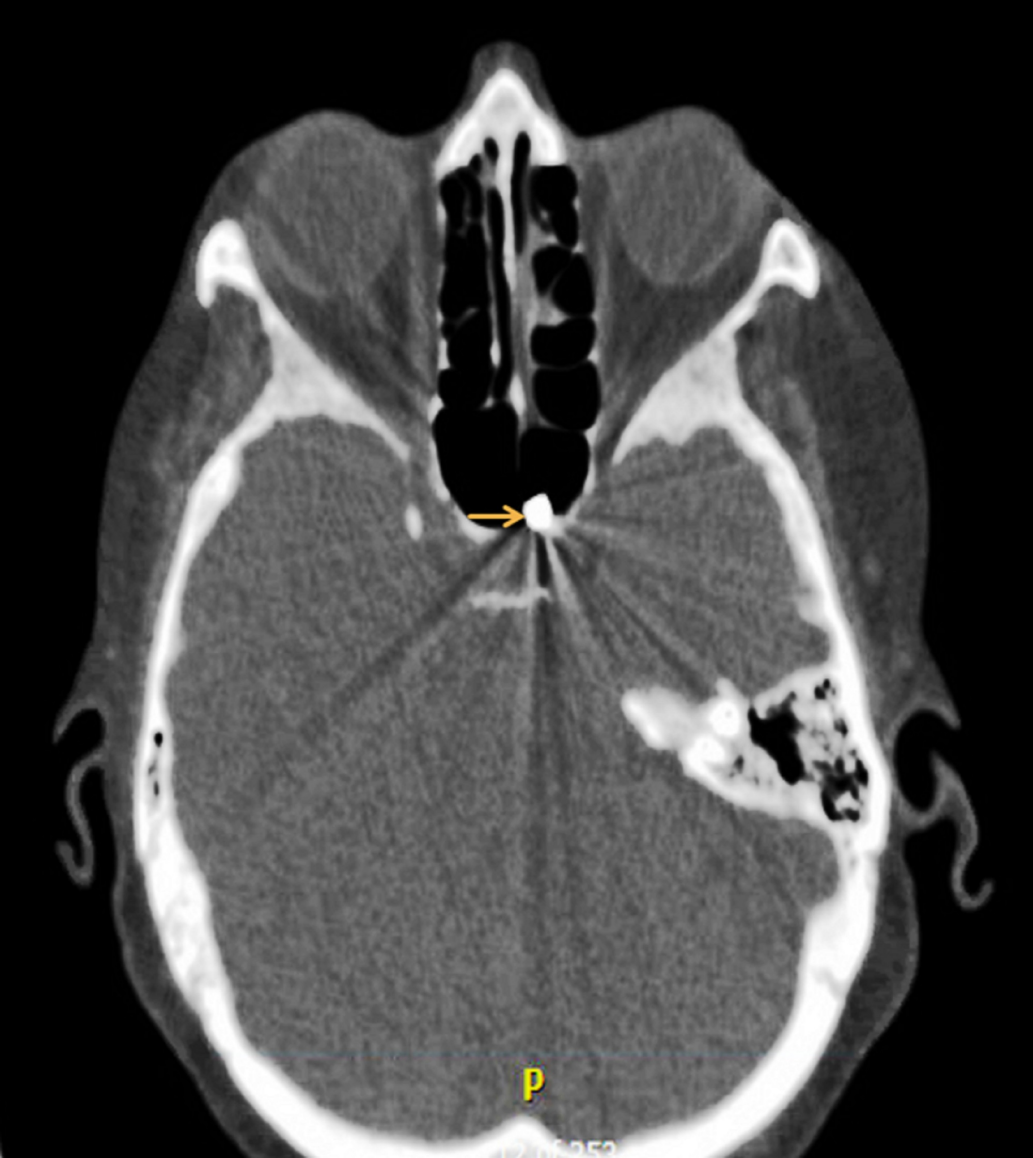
Computed tomography axial view at the level of paranasal sinuses showing the magnet with associated metallic artifact (arrow), originating from the left sphenoid sinus.
